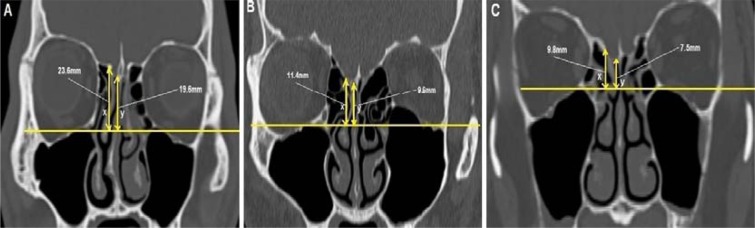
CT PNS coronal section showing the three types of TMS classification; (A) is Type 1 (low risk) where both OF-CP and OF-ER are 10 mm and above, (B) is Type 2 (moderate risk) where either OF-CP or OF-ER is less than 10 mm and (C) is type 3 (high risk) where both OF-CP and OF-ER are less than 10 mm.
